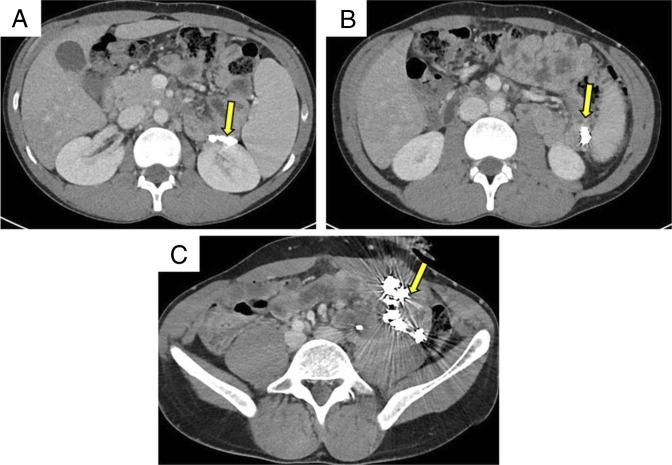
(A–C) Transverse sections of abdominal computed tomography showing embolization coils adjacent to (A) left kidney, (B) left retroperitoneum, and (C) left lower quadrant in the L. paracolic gutter (yellow arrow).
